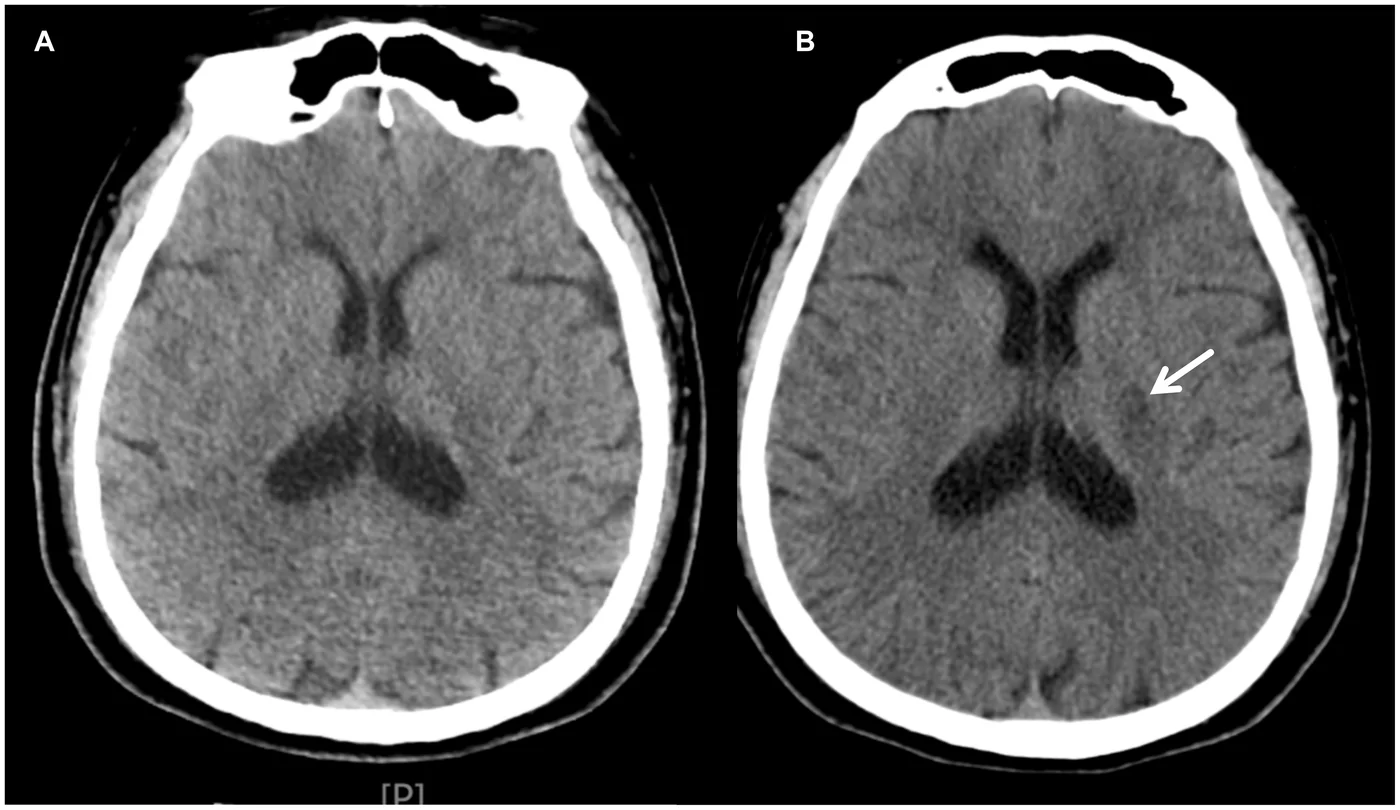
Serial non-contrast cranial computed tomography (CT) scans of the brain (axial views). (A) Initial non-contrast head CT at admission (3 h after symptom onset) shows a subtle focal hypodense area in the left basal ganglia region, without intracranial hemorrhage or mass effect. (B) Follow-up non-contrast head CT (48 h after symptom onset) demonstrates a well-demarcated hypodense infarct (white arrow) in the same left basal ganglia territory with increased lucency compared with the initial scan. Brain magnetic resonance imaging (MRI) could not be performed due to the patient's severe claustrophobia; the serial CT comparison confirms the location and evolution of the acute ischemic infarct, providing critical imaging evidence for the diagnosis and therapeutic decision-making of acute ischemic stroke.
